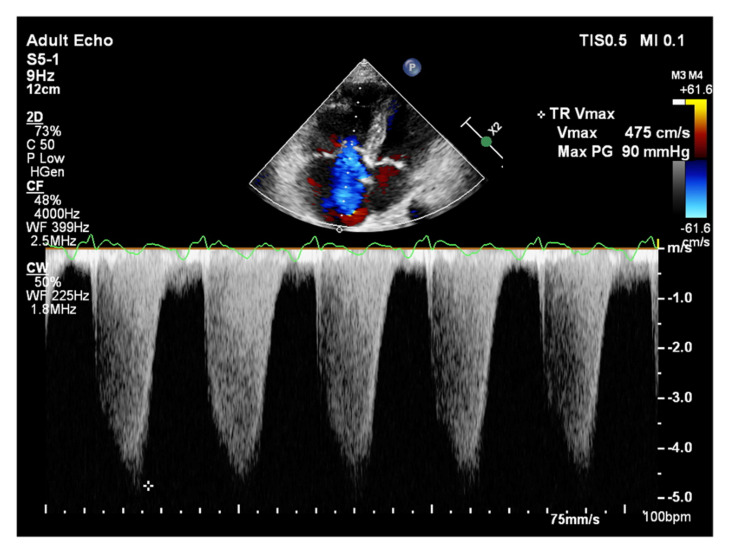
Doppler assessment of tricuspid regurgitant velocity (TRV). TTE, 4CH view. TTE: transthoracic echocardiography; 4CH: four-chamber.
